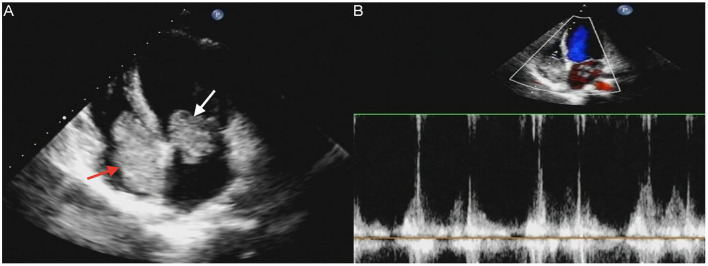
Transthoracic echocardiography (TTE) revealed masses attached to the atrial septum in the left atrial cavity (white arrow) and the right atrial cavity (red arrow) (A). Acceleration of tricuspid valves antegrade flow (B).
